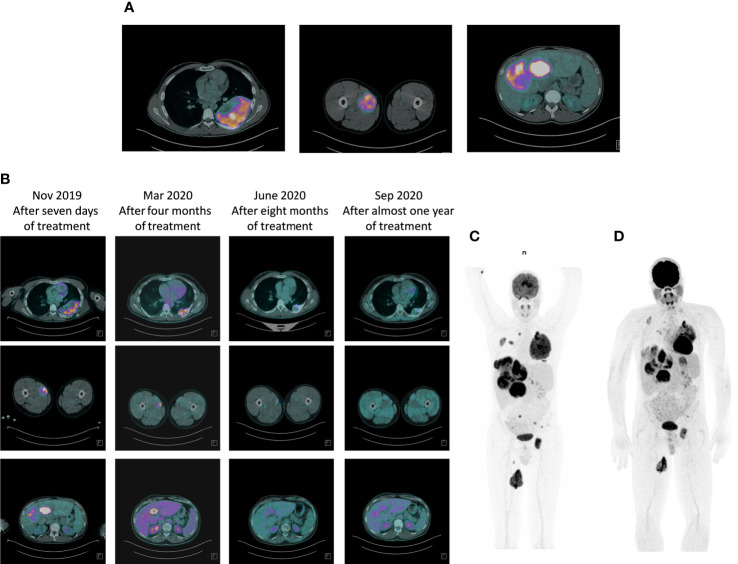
Response to therapy with larotrectinib: ^18^FDG-PET/CT scan evaluation. (A) Baseline FDG PET/CT study showing coronal fused PET/CT image with abnormal FDG uptake in multiple liver metastases, left rib and left femor. (B) Progressive metabolic response to larotrectinib after 7 days, four months, eight months and after almost one year of treatment. (C) Maximum intensity projection (MIP) PET/CT images: pre-therapy PET/CT scan again showed FDG-avid metastases occupying virtually the entire liver, left rib and left femor, all demonstrating high FDG avidity. (D) Seven days after the start of larotrectinib, PET/CT scan showed early tumor shrinkage with a reduction in FDG avidity in all previously described lesions.
